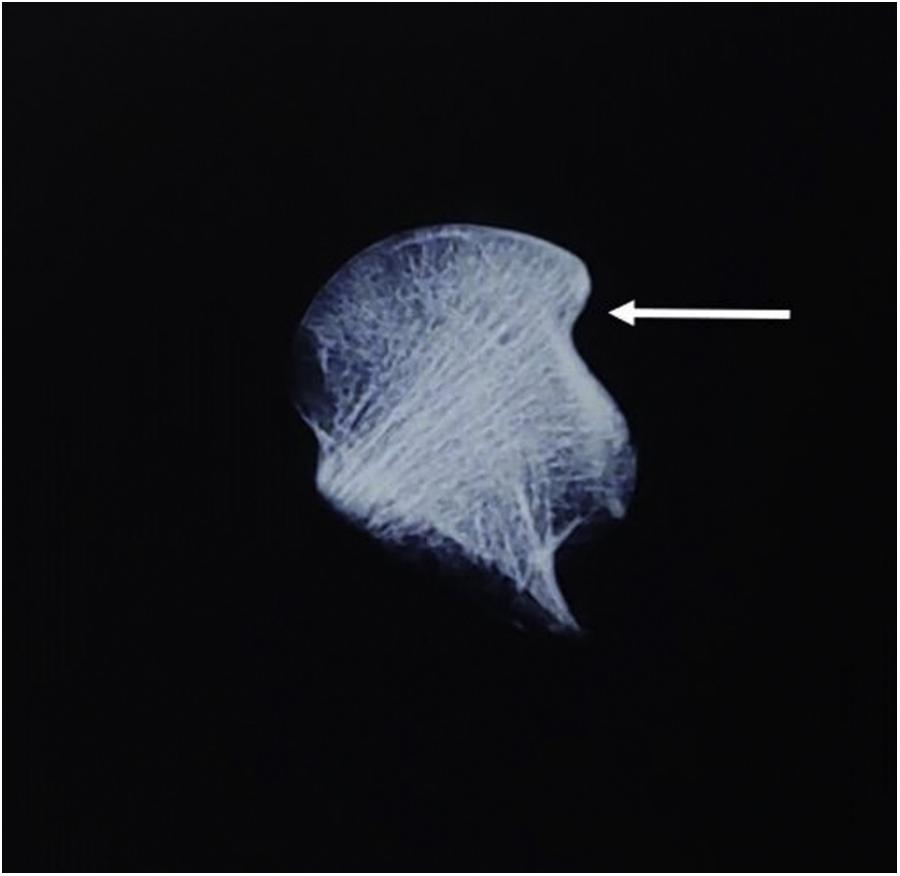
Radiograph of the excised femur head without any signs of osteonecrosis. Indentation is seen on the superolateral aspect (arrow).
